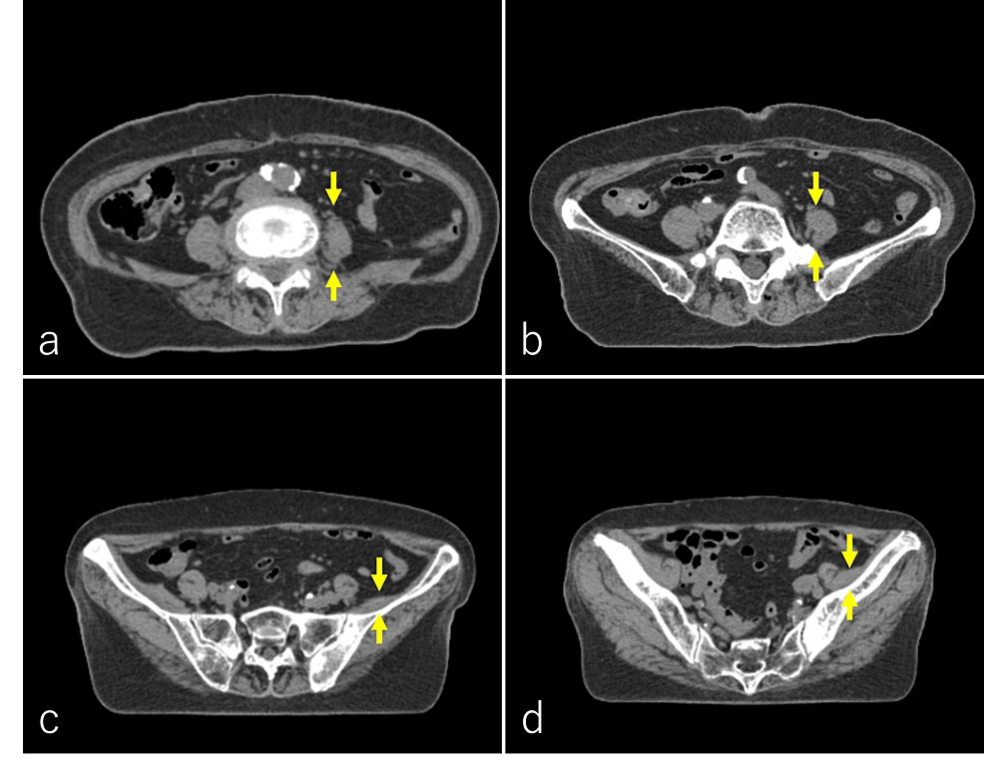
Axial pelvic CT before radiation therapy. CT images of the pelvic region revealed atrophy of the left psoas muscle compared to the right psoas muscle (a-b, yellow arrows). Some atrophy of the left iliacus muscle was also present (c-d, yellow arrows).
